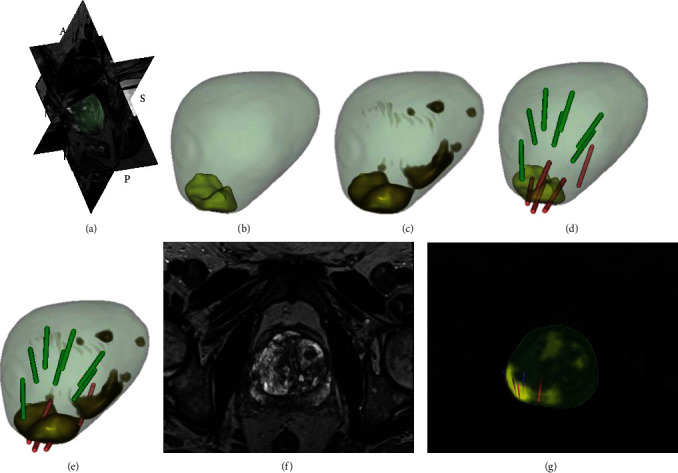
An example of model detection: a 70-year-old man in the testing set with prostate-specific antigen of 30.2 ng/mL and PI-RADs of 5. (a) The patient's prostate is visualized in 3D coordinates for volume representation. (b) The patient's prostate surface and its ROI (yellow) outlined by radiologists. (c) The patient's prostate surface and its potential cancer distribution (dark yellow) generated by the WSUNet. (d) The patient's original ROI (yellow) and each biopsy core (Gleason grade ≥ 7 marked as red and <7 masked as green). (e) The ROI (dark yellow) detected by the model and each biopsy core (Gleason grade ≥ 7 marked as red and <7 masked as green). (f) A slice of the T2-weighted prostate MRI. (g) Slices with the detected ROI (brilliant yellow) and the positive biopsy core (red). In this meaningful example, targeted biopsy yielded no meaningful results, and the model presented a cancer distribution, part of which was validated by systematic biopsy. In this example, the model successfully detected MIPCas, which radiologists have not outlined.
